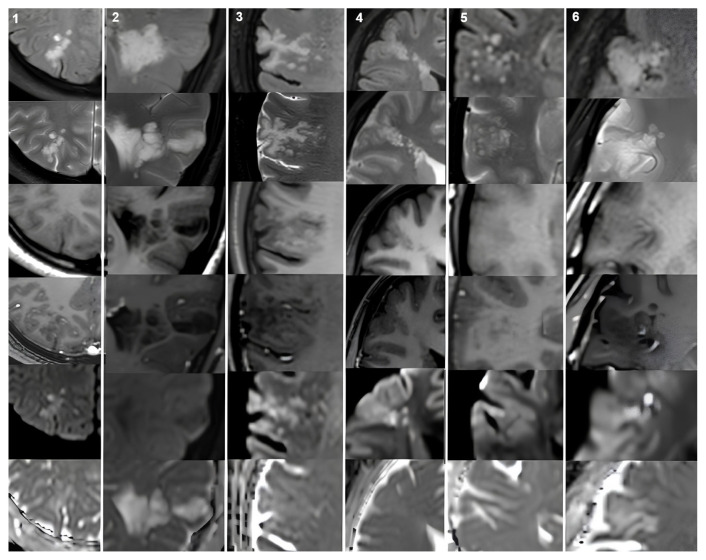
Representative MVNT cases. Each column contains MRI images of different cases. All the lesions are hyperintense on FLAIR and T2WI (first and second row). On precontrast T1WI, all the lesions are isointense compared to adjacent parenchyma except case 2. The MVNT lesion in case 2 has a hypointense signal on T1WI (third row). None of the lesions show contrast enhancement on postcontrast T1WI (fourth row). All the lesions except case 2 are hyperintense on b1000 DWI. The lesion in case 2 has an isointense signal compared to the adjacent parenchyma on DWI (fifth row). No hypointensity was observed on the ADC maps in any of the lesions (sixth row). In terms of the nodularization pattern, cases 1 and 4 had multiple small patterns, case 2 had multiple large patterns, and the remaining cases had a mixed pattern.
